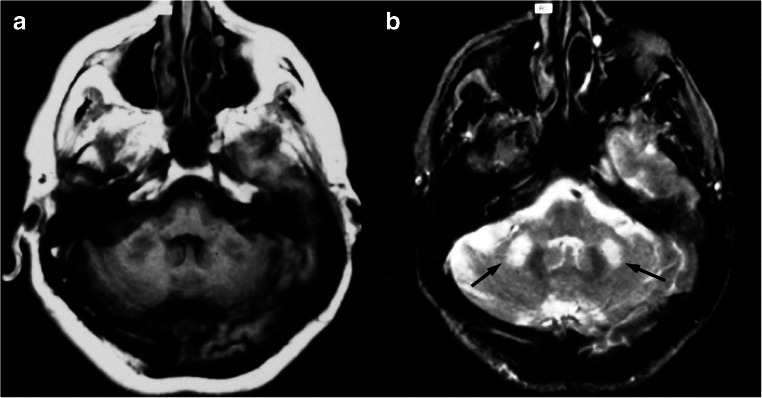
Imaging findings in a 55-year-old male patient with FXTAS. Axial T1- (a) and T2- (b) weighted sequences show the presence of a mild global cerebellar atrophy, associated with the typical T2-weighted hyperintensity of the middle cerebellar peduncles (black arrows in b)
